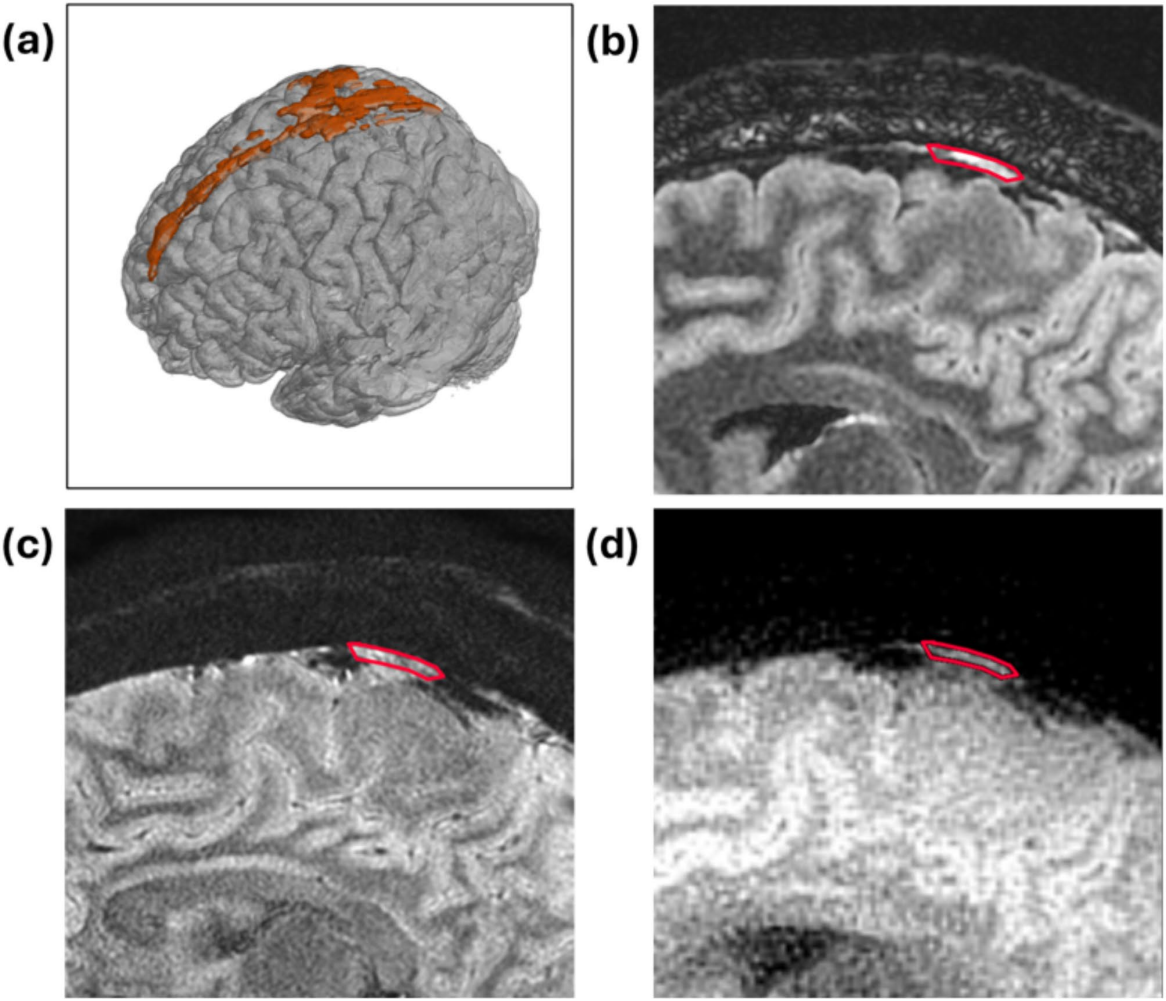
(a) 3D FLAIR reconstruction showing the PSD structure in orange, (b) 2D T2 FLAIR image, (c) MUSE DWI, and (d) PROP DWI. The red contour outlines the PSD region, as identified on DWI, corresponding to the high signal in the PSD on FLAIR images. This overlay enhances geometric accuracy visualization and highlights the improved delineation of the PSD structure using MUSE and PROP techniques.
